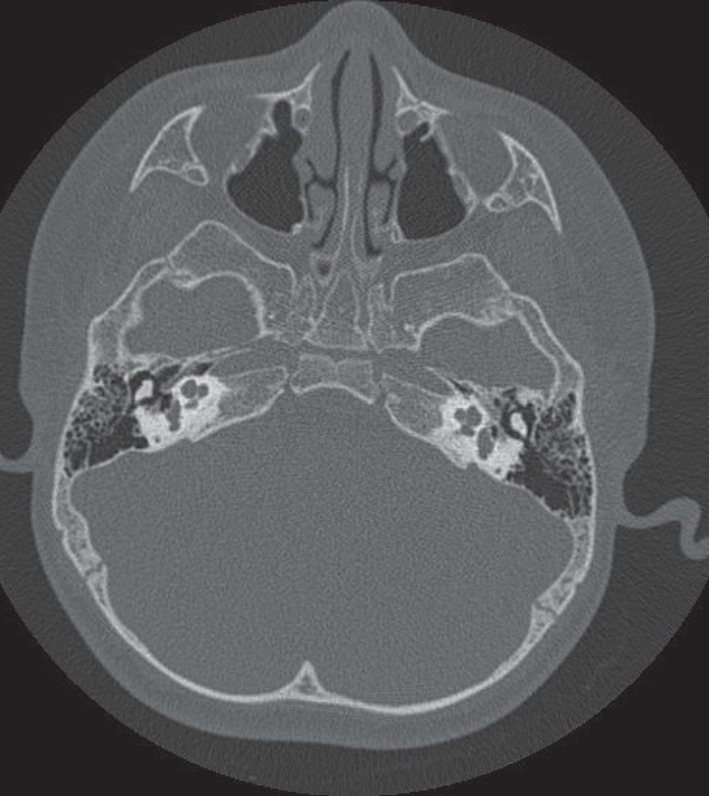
CT scan of the temporal bone with incomplete partition (IP-2).
